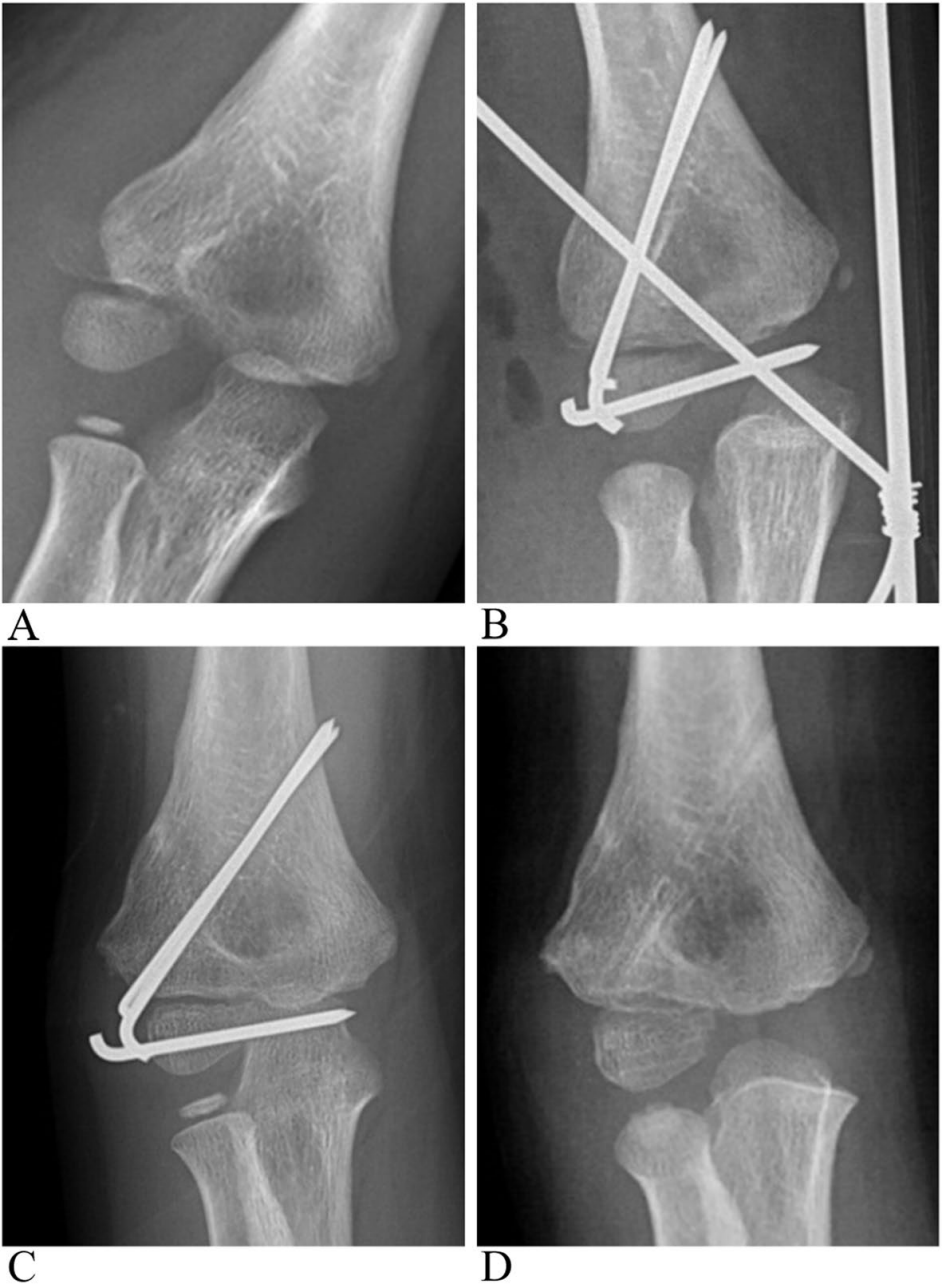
A 5 years old boy with a Song stage 4 and Milch typeILCHF treated with ORIF directly. A Pre-operative anterior-posterior X-ray. B post-operative X-ray showed nearly anatomic reduction. C 5 months post operation showed well fracture union but a decreased Baumann angle. D X-rays after internal pins’ removal
